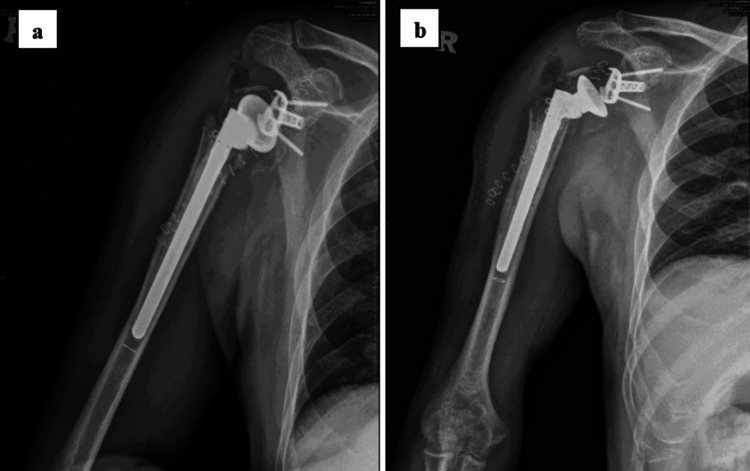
Post-operative radiographs of the right shoulder reveal a secure fixation of reverse shoulder arthroplasty (Implantcast™, Germany) with adequate cement mantle implantation and a radiolucent glenoid hemisphere. Implant used (Implantcast™, Germany). Cementless right glenoid baseplate anatomical size 2. Glenoid hemisphere cap size 40mm. Right cemented humerus shaft stem size 10mm in diameter and 120mm in length. Two cancellous peripheral screws are 4.2mm and 34mm in length. One central peg screw is 6.5mm and 25mm in length. (a) Lateral view; (b) Anteroposterior view
